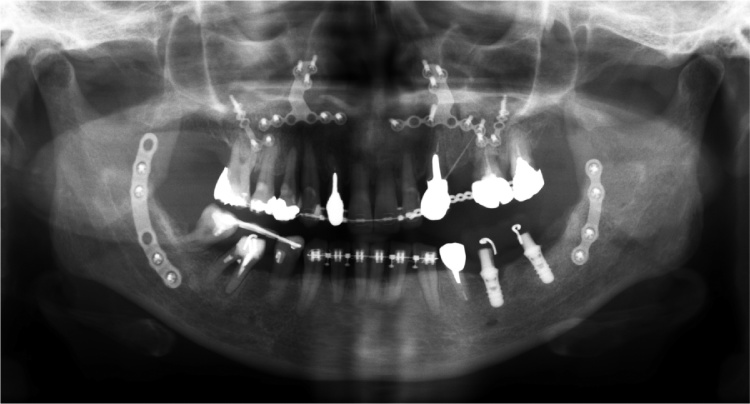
Panoramic X-ray at 6 months after the surgery in Case 2.
